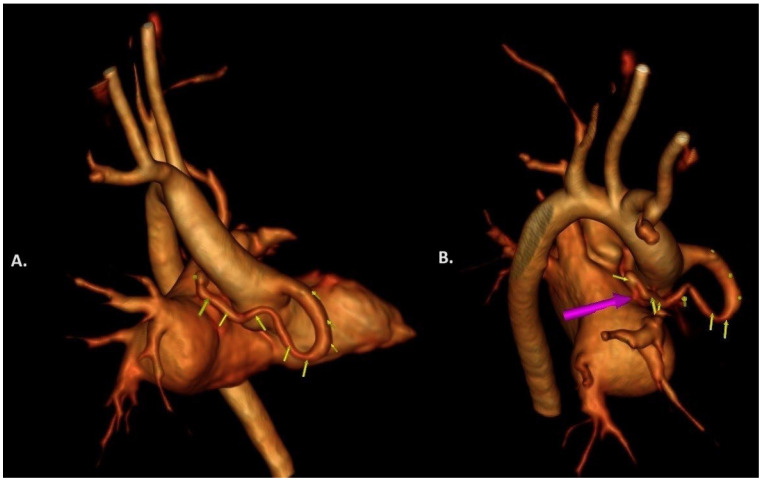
CT angiography 3D reconstruction highlighted a tortuous type B right coronary artery fistula (A) to the left atrium (yellow arrows) and the drainage place (pink arrow) (B).
